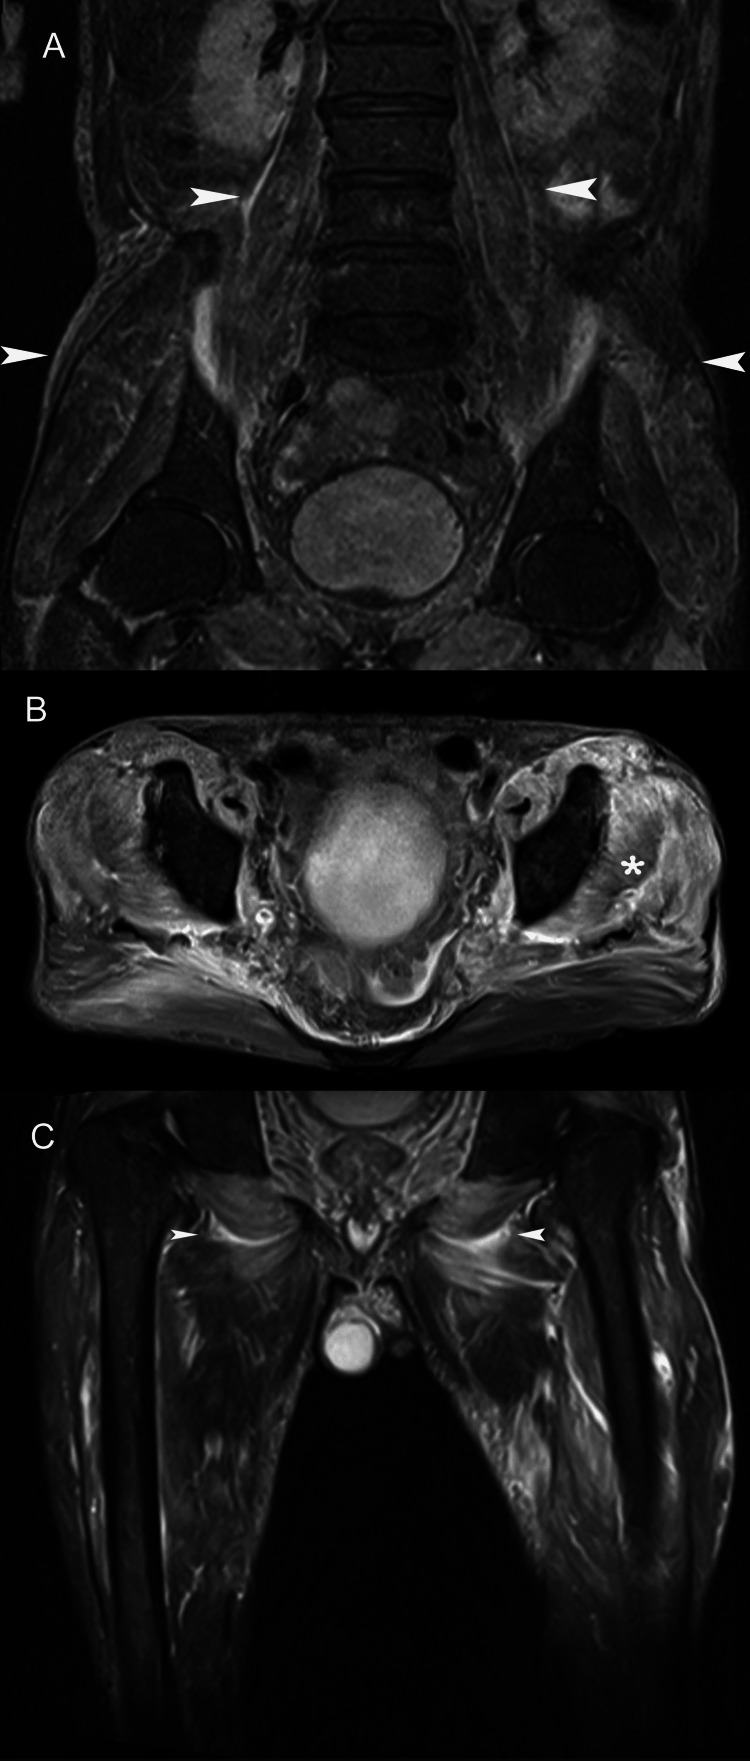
A 52-year-old man presented with weakness, malaise, and painful swelling of the trunk and thighs. Muscle biopsy disclosed nonspecific (idiopathic) myositis, and the patient was started on steroids with rapid clinical improvement. (A) Coronal fat-suppressed fast spin-echo T2-weighted MR image shows diffuse edema in psoas muscles and pelvic girdle musculature (arrowheads). (B) Axial short-tau inversion recovery MR image through the pelvis shows diffuse increased signal in enlarged gluteal muscles (asterisk). (C) Coronal fat-suppressed fast spin-echo T2-weighted MR image shows diffuse edema in the adductor magnus and peritrochanteric muscles.  Perifascial fluid is seen between the obturator internus and externus muscles (arrowheads).
